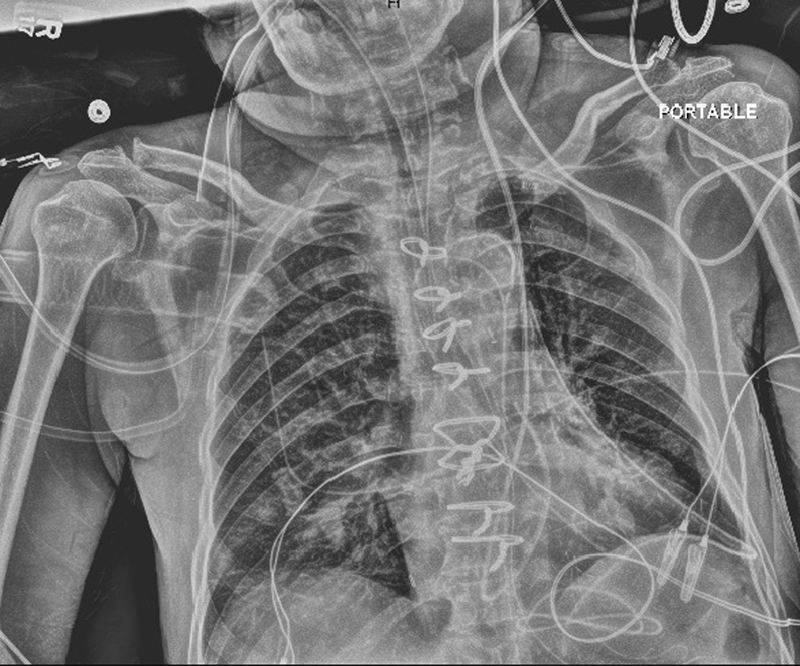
Postoperative chest radiograph demonstrating the abnormal course of the pulmonary artery catheter as it exits the coronary sinus and passes through the tricuspid valve into the right ventricular and the pulmonary artery.
